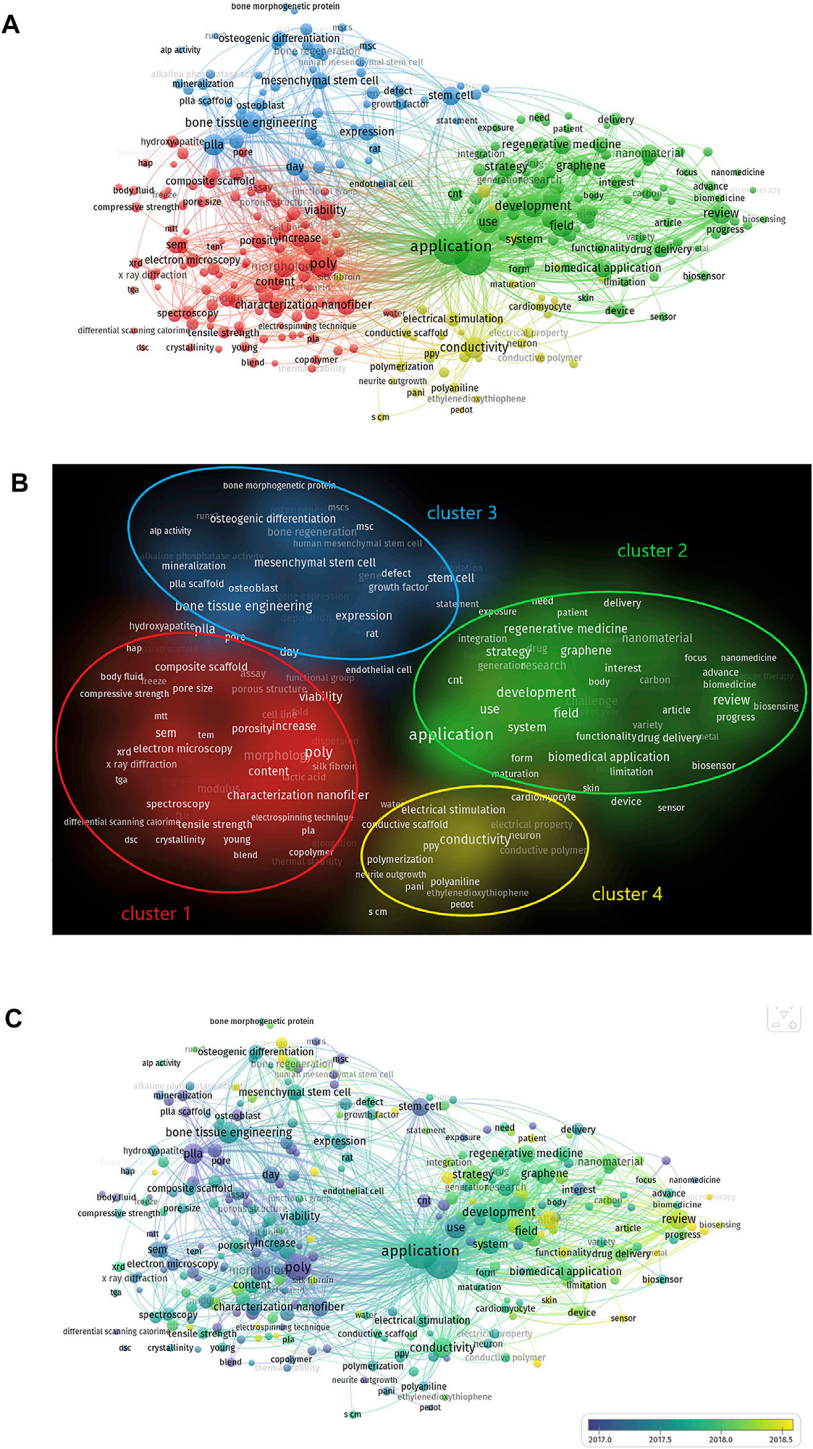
The analysis of keywords in publications on electroactive biomaterials used in TE. (A) Mapping of keywords in the field of the use of ultrasound in emergency medicine. The size of the circle represents the frequency with which keywords appear. (B) Mapping of the keywords in the area of electroactive biomaterials used in TE. The words were divided into four clusters in accordance with different colours that were generated by default, specifically, characterization of electroactive materials (lower left in red), function of electroactive materials (upper right in green), application of electroactive materials in bone tissue engineering (upper left in blue) and application of electroactive materials in nerve and cardiac tissue engineering (lower right in yellow); (C) The distribution of keywords is presented according to the average time of appearance. The blue colour represents an early appearance, and the yellow colour represents a late appearance.
